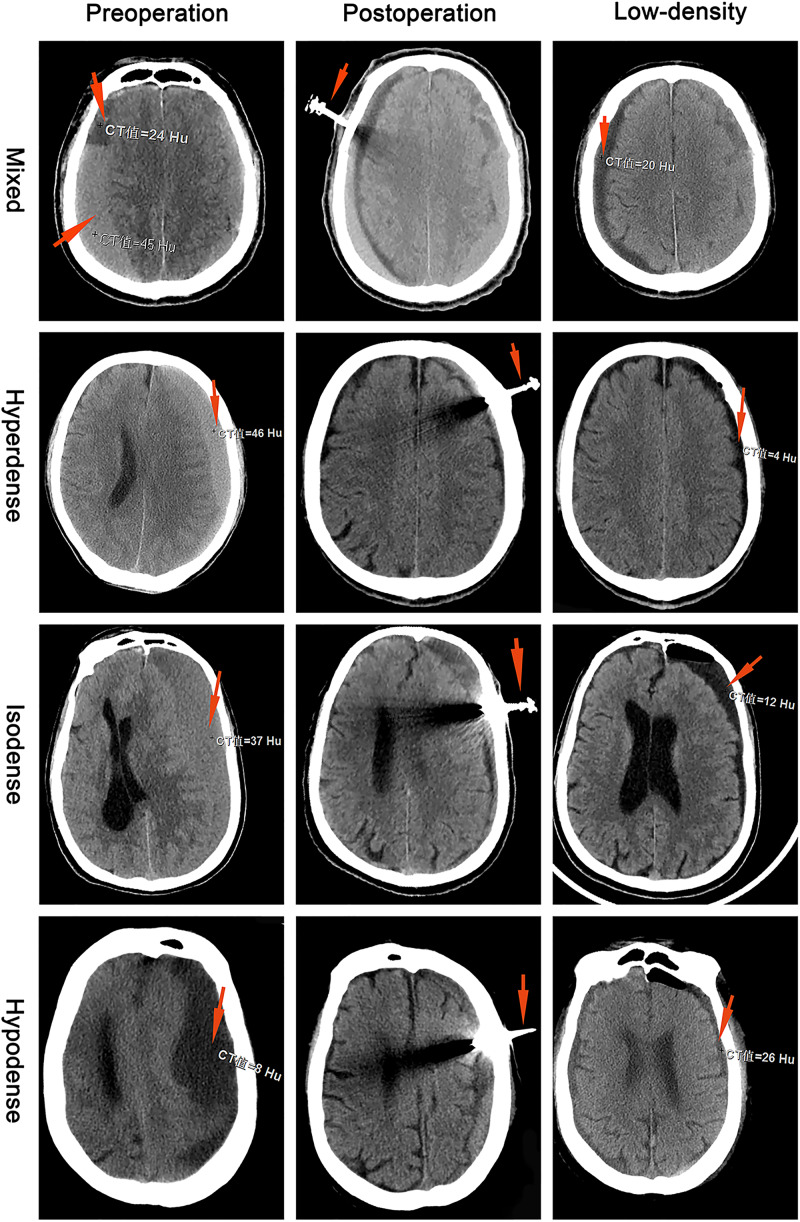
Chronic subdural hematoma classification: mixed, hyperdense, isodense, and hypodense. The preoperative red arrows show the CT values (Hu) for different CSDH types. The postoperative red arrow shows the YL-1 drainage tube. The low-density red arrow shows that the CT value (Hu) of the CSDH was less than 30.
